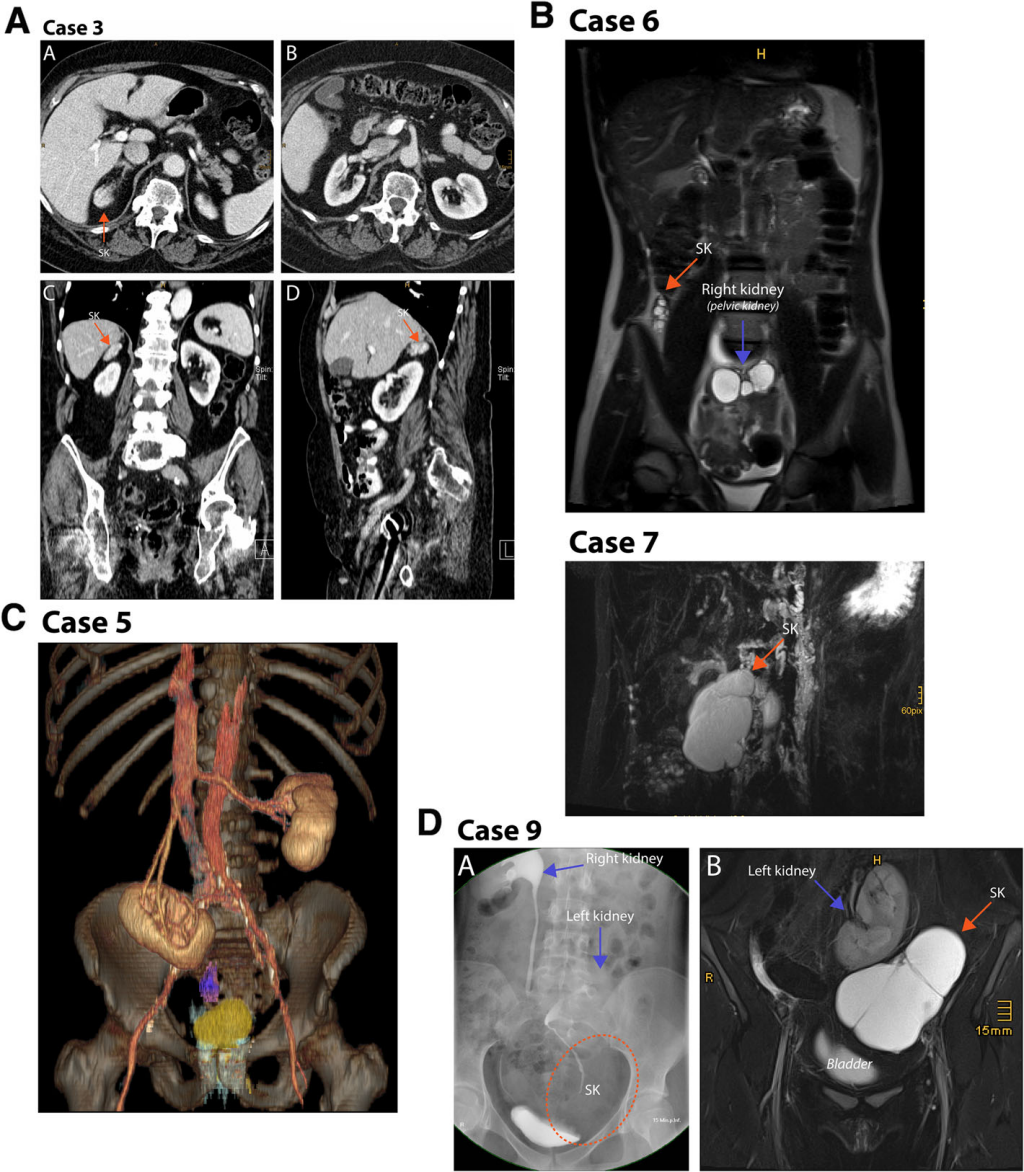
Case 3: (a) Transversal contrast-enhanced CT of a supernumerary kidney (SK) on the right, located dorso-lateral and at the same level as the ipsilateral adrenal gland. The SK presents with the same attenuation as the contralateral kidney. b Transversal contrast-enhanced CT of the normal kidneys bilaterally. c Sagittal CT-reconstruction. SK located cranial to the normal kidney. d Coronal CT-reconstruction with SK located cranial to the normal kidney. Case 5: Coronal 3D volume rendering reconstruction of a contrast-enhanced CT, SK in violet, bladder in yellow. Case 6: T_2_-weighted coronal image of the abdomen, showing the supernumerary kidney and the hydronephrotic right kidney (pelvic kidney). Case 7: T_2_-weighted thick-slab semi-coronal image of the supernumerary kidney and the normal kidney. Case 9: (a) T_2_-weighted coronal slice of the left kidney, the SK and bladder. (B) Intravenous pyelogram (15 min after i.v. contrast administration) depicting the right and left kidney as well as the supernumerary kidney as a mass and bladder
